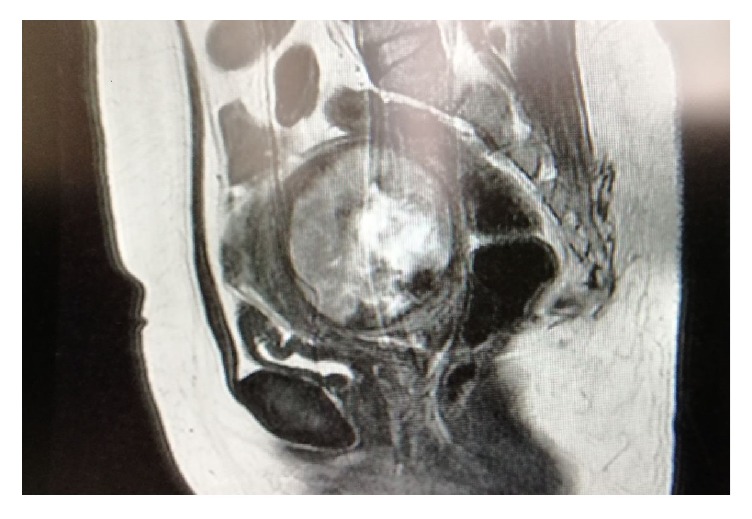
MRI image of myoma before surgery.
